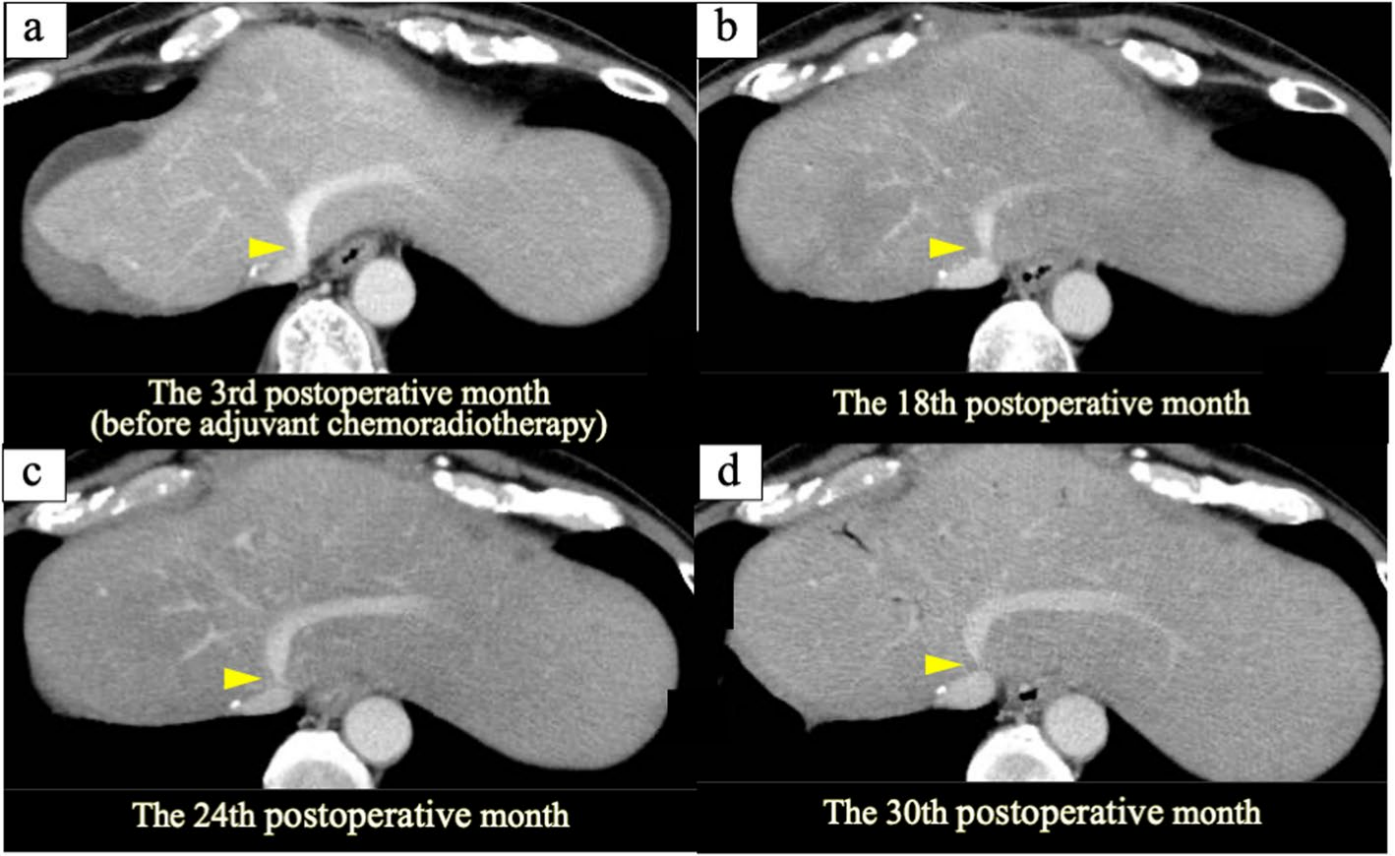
Multidetector-row computed tomography findings of the gradual narrowing process of the left hepatic vein. Yellow arrowheads show the root of the left hepatic vein. a The 3rd postoperative month. b The 18th postoperative month. c The 24th postoperative month. d The 30th postoperative month
